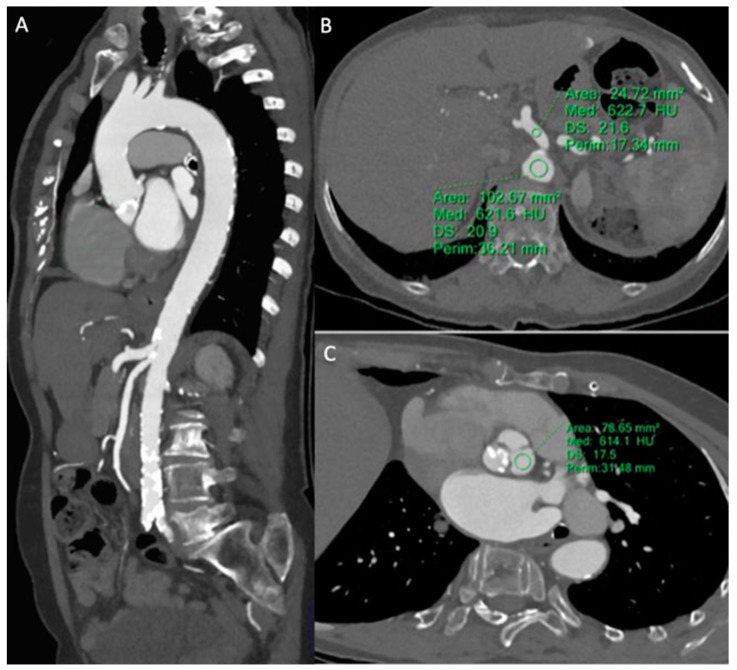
Pre-TAVR CTA with the MBIR algorithm—MIP parasagittal (A) reconstruction of the entire aorta. An optimal and homogeneous arterial opacification was obtained at each different aortic level with a low contrast medium volume of 60 mL and an intra-vessel density of up to 622 HU in the abdominal aorta at the celiac trunk level (B) with low noise values of 13 and 21 HU, respectively. (C) CT reconstruction of the aortic bulb correctly shows calcifications of the non-coronary aortic sinus and a high attenuation value of 614 ± 17 HU.
